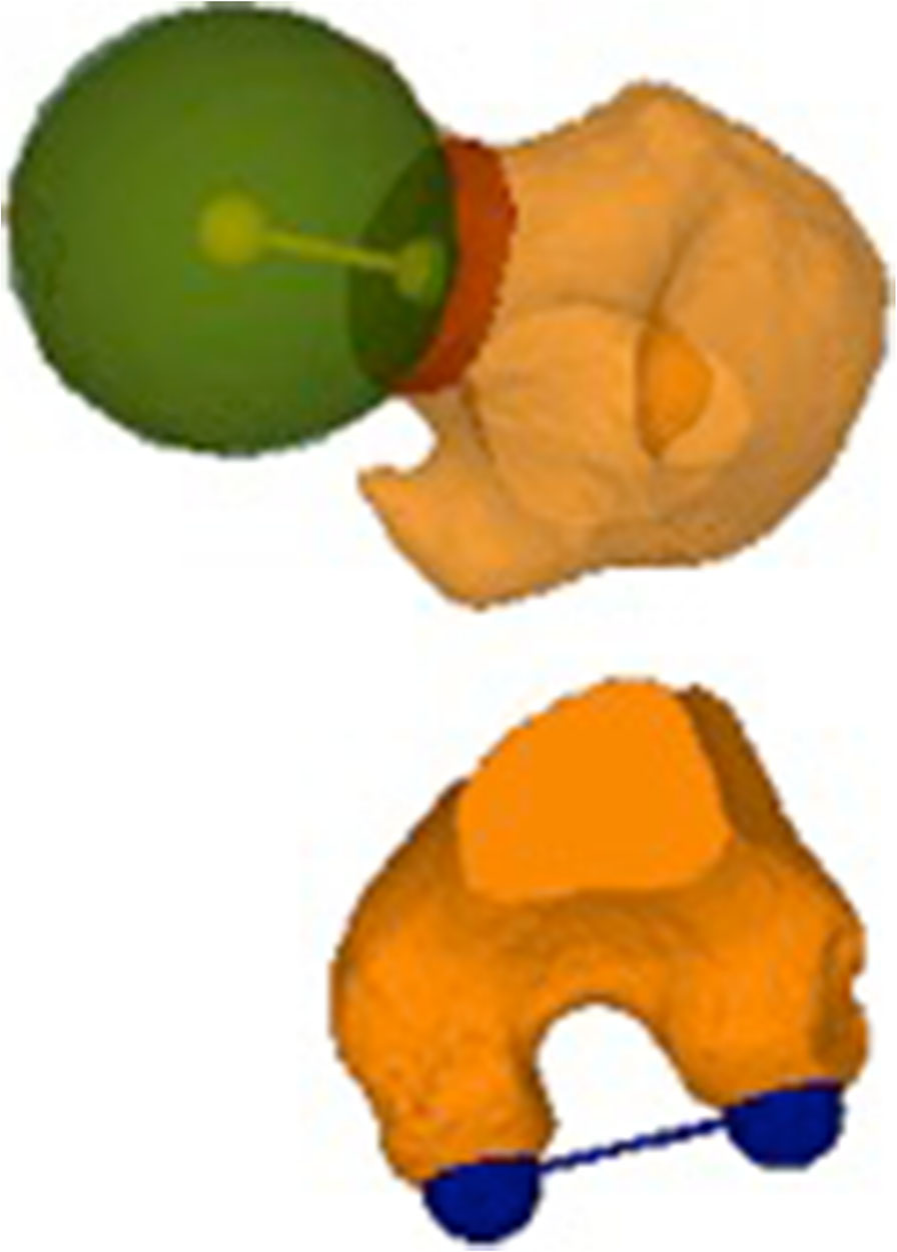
Femoral condylar axis (blue) and femoral neck axis (yellow) of a CT rendered image. The figure was created by the authors using the in-house developed surgical planning software CASPA (Balgrist CARD AG, Zurich, Switzerland)
